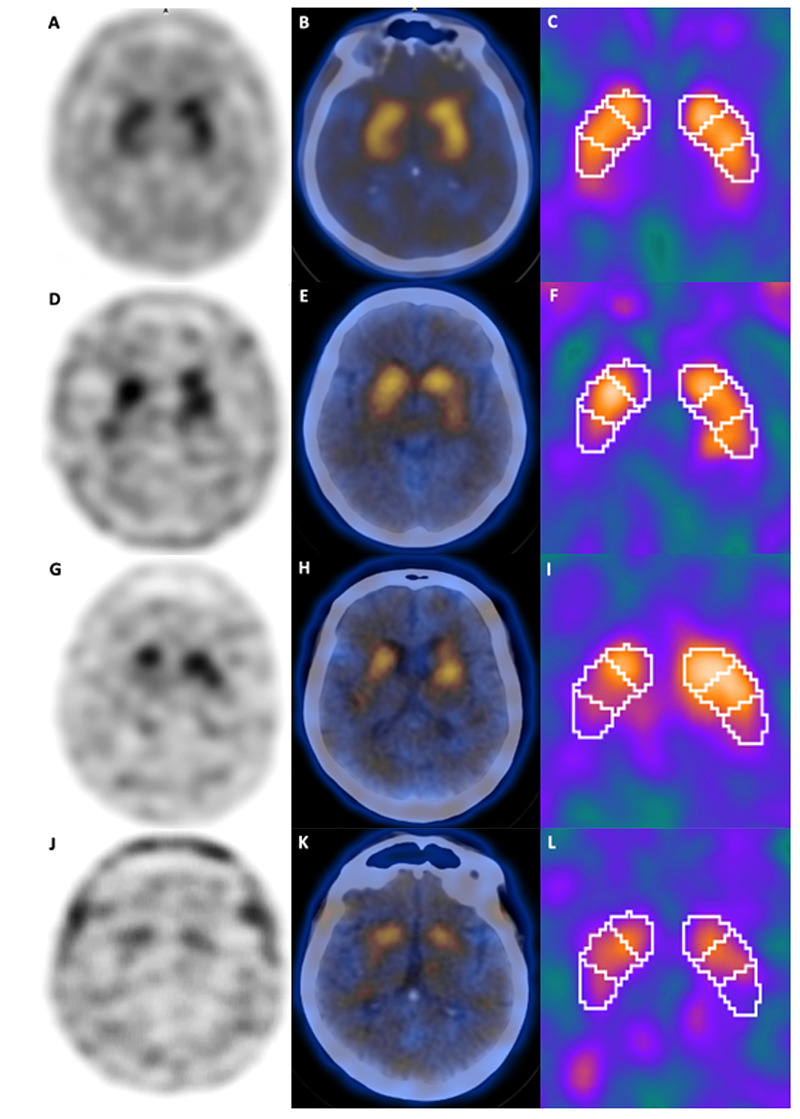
DAT-SPECT from healthy controls and from PD patients at different stages of the disease. A standard DAT-SPECT imaging appears as two intense symmetric “comma-shaped” regions of activity in the striatum (A, B,C). With the progression of PD, there is a decline in radiotracer uptake that follows a gradient from posterior to anterior structures, as shown through DAT-SPECT obtained during early (D,E,F), moderate (G,HI) and late (J,K,L) stage of PD.
